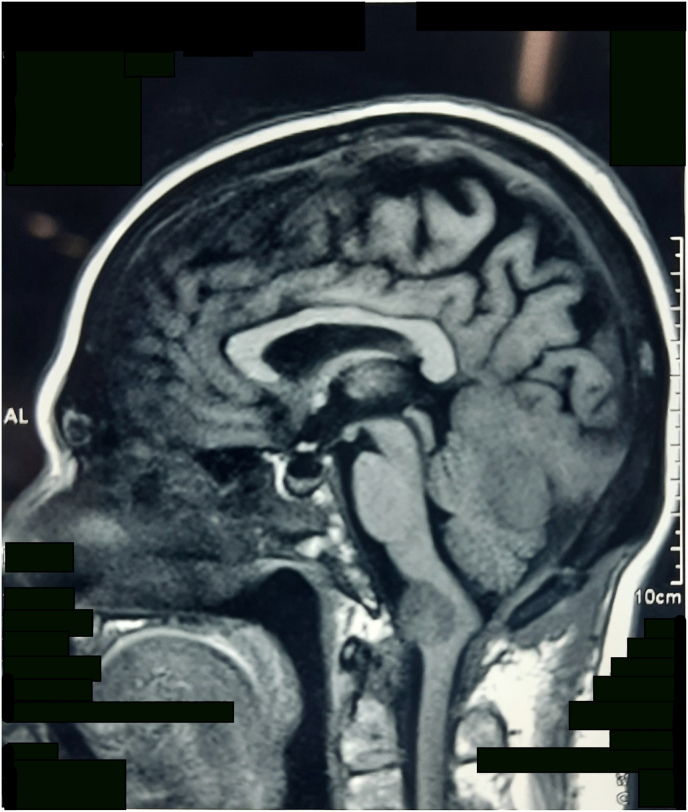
Post-surgery MRI brain shows no significant interval change in the sizes of extra-axial mass adjoining the cerebellar hemisphere and medulla.
